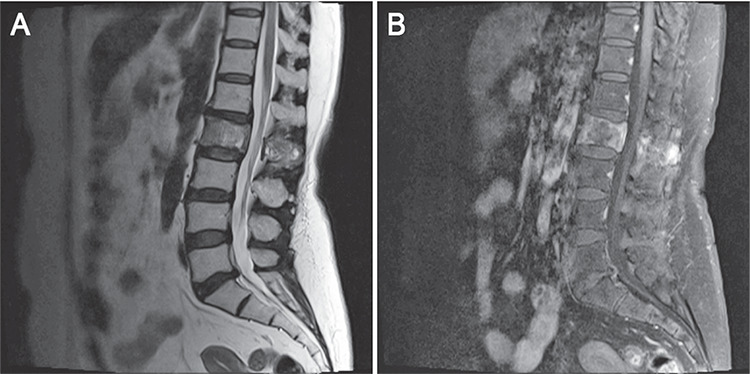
T2-weighted lumbar magnetic resonance imaging (MRI) images depicted diffuse heterogeneous density changes (A) and contrast-enhanced T1-weighted MRI images showed contrast enhancement involving the body of the second lumbar vertebra that was extending to the posterior elements, and reported as suspicion for osseous metastasis (B)
